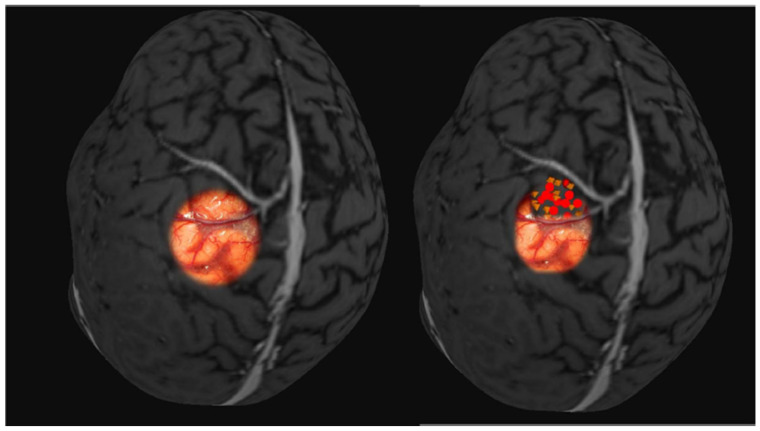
Three-dimensional MRI with points obtained in motor response in TMS (yellow octahedrons), and motor response recorded in the DES mapping (red circles) during the surgery. Fusion with intraoperative imaging. DES: direct electrical stimulation; MRI: magnetic resonance imaging; TMS: transcranial magnetic stimulation.
